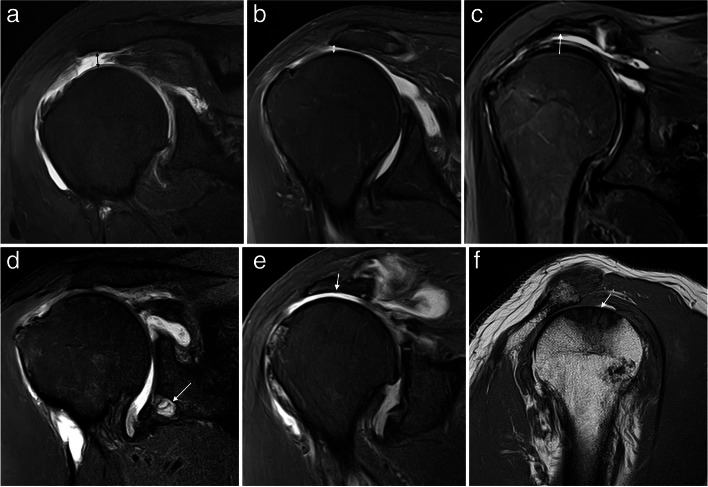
Hamada rotator cuff arthropathy evolutive stages. a–c Oblique coronal fat-suppressed PD-weighted MRI. Stage 1 showing an acromiohumeral interval (AHI) > 5 mm (double-headed arrow in a). Stage 2 the AHI is < 5 mm (double-headed arrow in b). Stage 3 is same as stage 2 but with the acetabularization of the acromion undersurface (arrow in c). d, e Oblique coronal fat-suppressed PD-weighted MRI. Stage 4a is similar to stage 2 but with degenerative changes in the glenohumeral joint (arrow in d). Stage 4B adds acromion acetabularization (arrow in e). f Sagittal oblique T1 MRI. Stage 5, there are osteonecrotic changes in the humeral head vertex (arrow in f)
